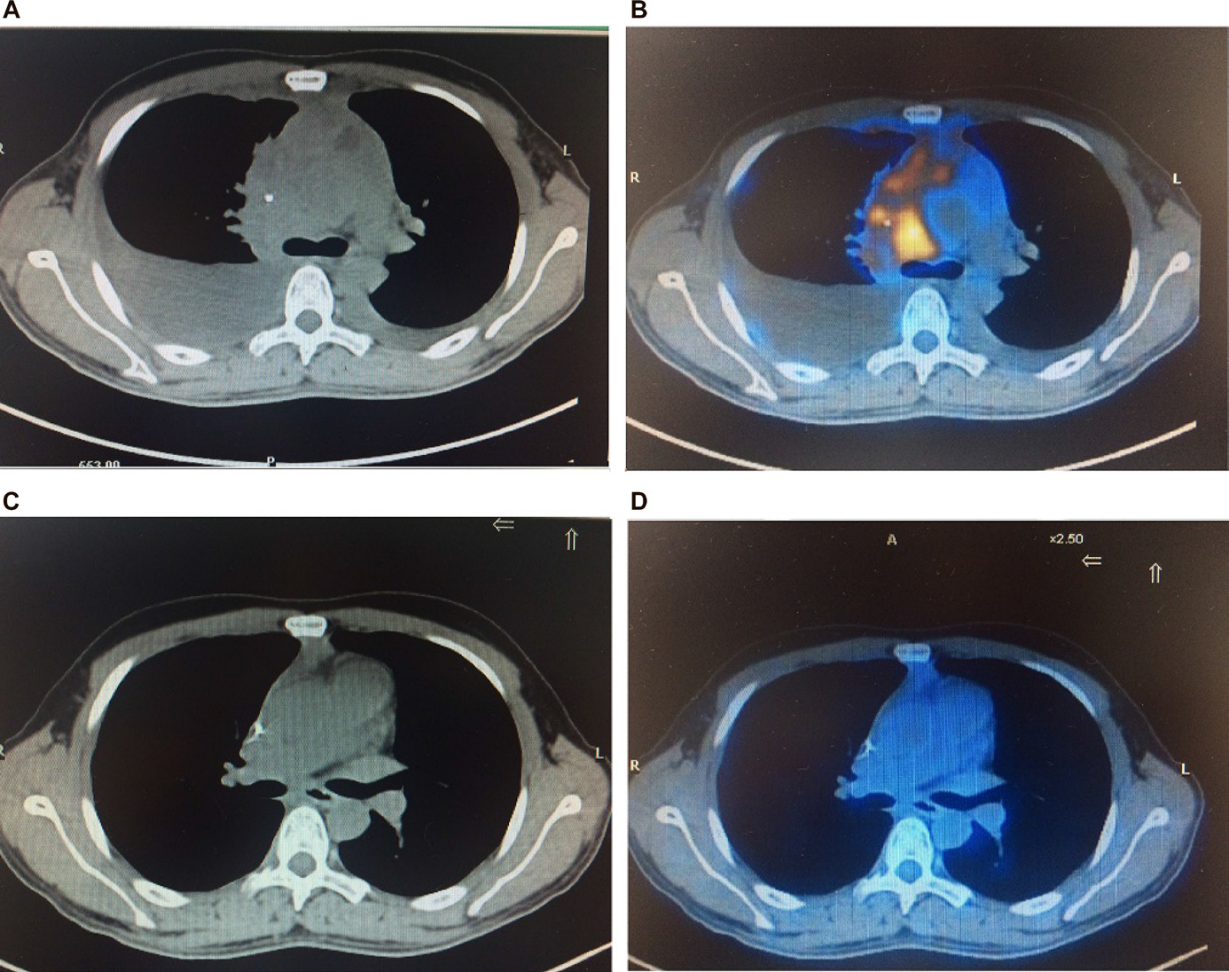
Baseline and interim PET-CT scans for a patient of T-LBL Baseline CT (A) and PET (B) scan revealed a mediastinal mass and moderate pleural effusion. Interim PET-CT scan (C and D) after induction therapy confirmed CR.
